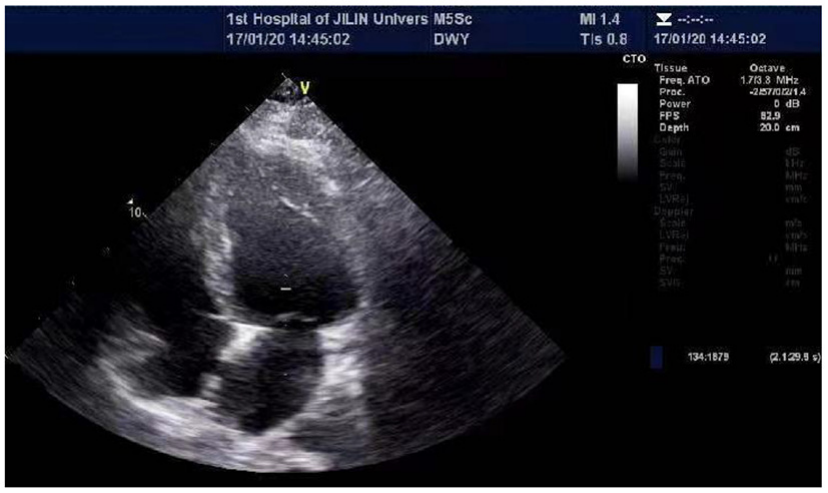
Echocardiographic of the patient's heart. The echocardiography revealed EF 29%, left atrial and left ventricle enlargement (left ventricular endomesosomal diameter 63 mm), reduced ventricular wall pulsation diffusion, decreased left ventricular systolic and diastolic function, and trace pericardial effusion.
